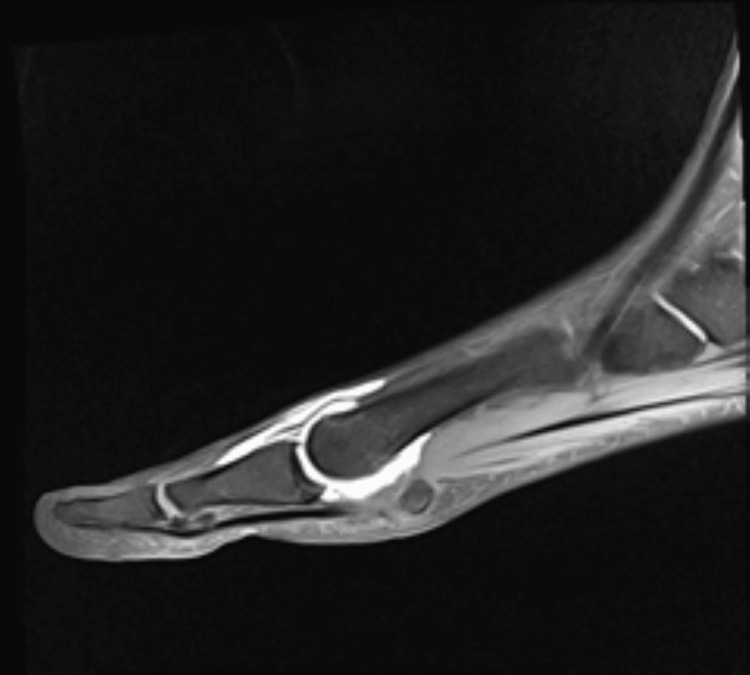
MRI scan with contrast of the great toe metatarsophalangeal joint showing rupture of the capsular ligamentous complex MRI arthrogram of a 32-year-old male patient presenting with hyperextension injury of the great toe.
